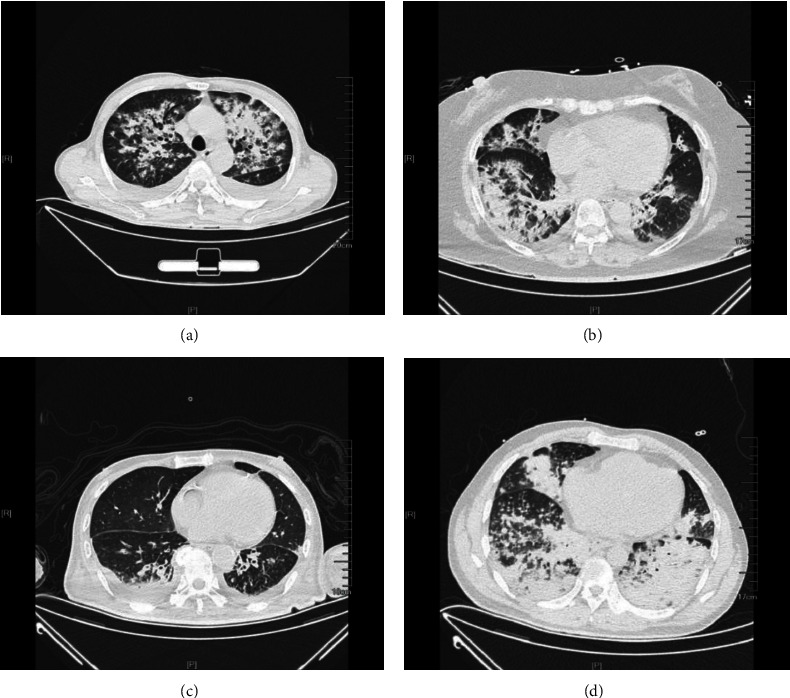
Chest CT examination of common bacterial infection with Gram negative (positive) bacteria. (a) *Staphylococcus aureus* infection. (b) *Klebsiella pneumoniae* infection. (c) *Pseudomonas aeruginosa* infection. (d) *Escherichia coli* infection.
